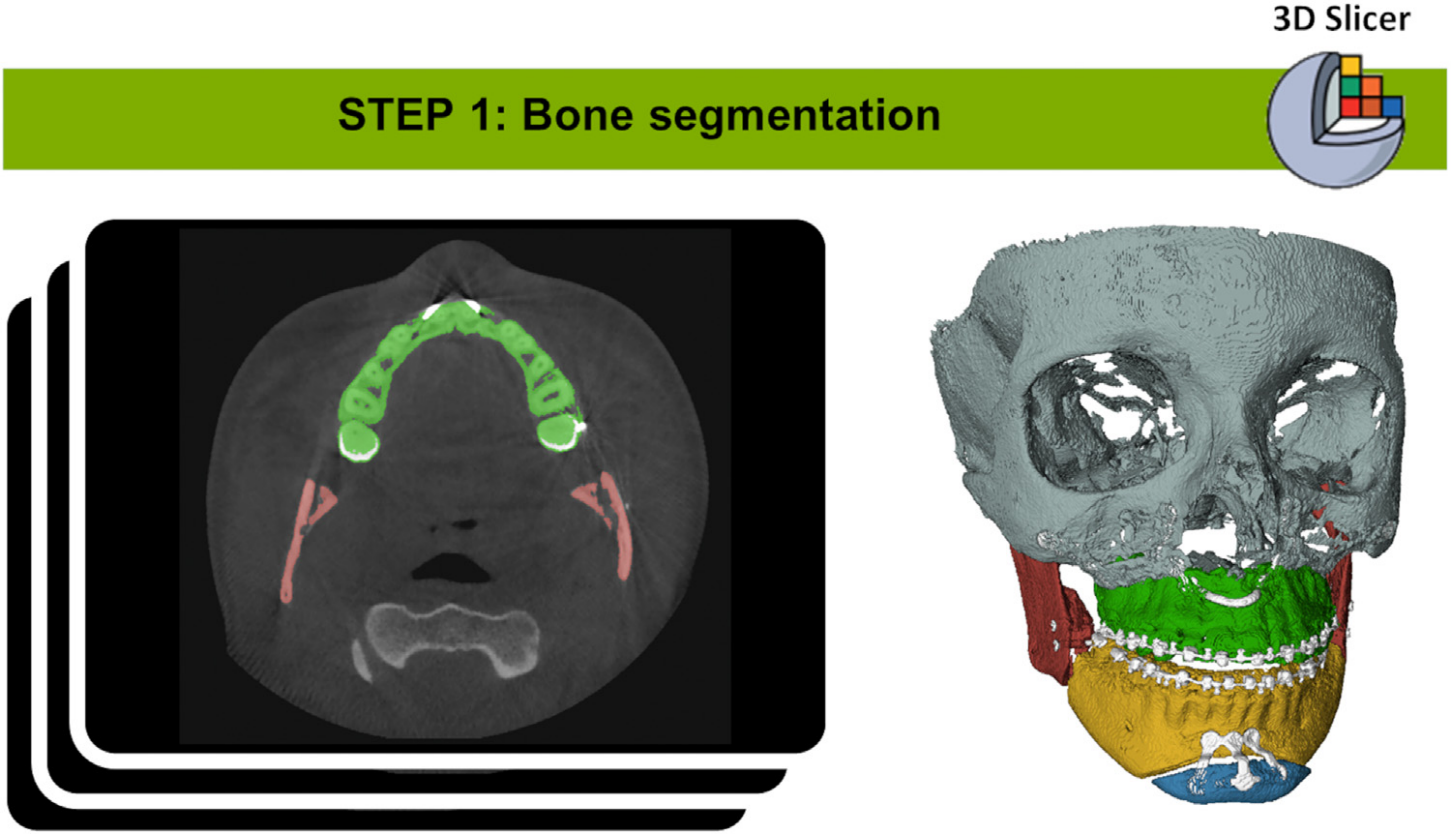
Bone segmentation of post-operative CT Images by using the *Segment Editor* module of 3D Slicer software.
